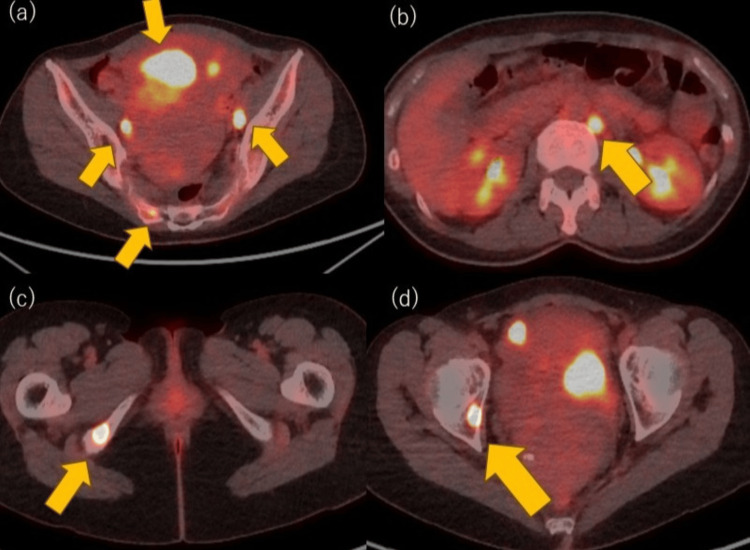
PET-CT images demonstrating multiple areas of increased uptake throughout the body: (a) uterus, pelvic lymph nodes, and sacrum; (b) para-aortic lymph nodes; (c) ischium; (d) acetabulum (yellow arrows) PET-CT: Positron emission tomography-computed tomography
